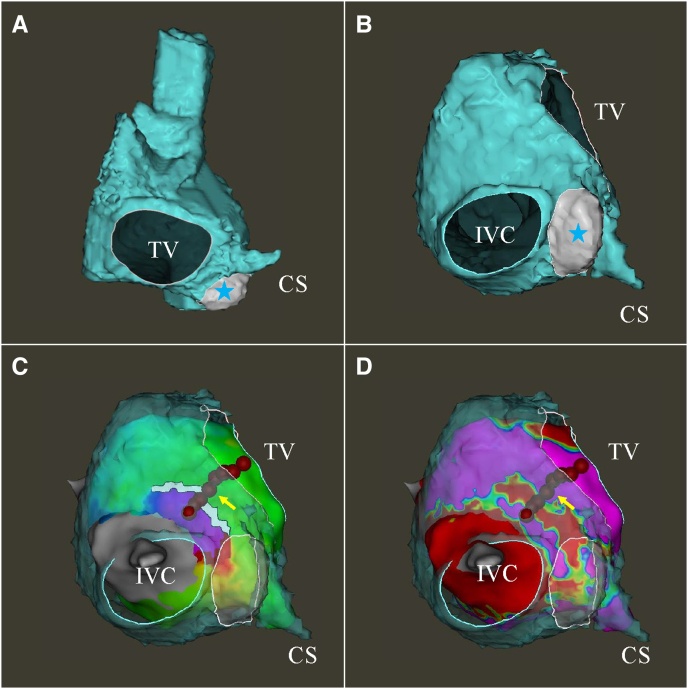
Three-dimensional construction of computed tomography. (*A*) Left anterior oblique view. A pouch with a depth of 11.1 mm (blue star) was identified in the septal side of the cavotricuspid isthmus. (*B*) Inferior view. (*C*, *D*) The tachycardia circuit and the low voltage area were anatomically adjacent to the edge of the pouch detected by computed tomography. The termination of tachycardia was achieved at the boundary site (yellow arrow). Abbreviations as in *Figure 2*.
